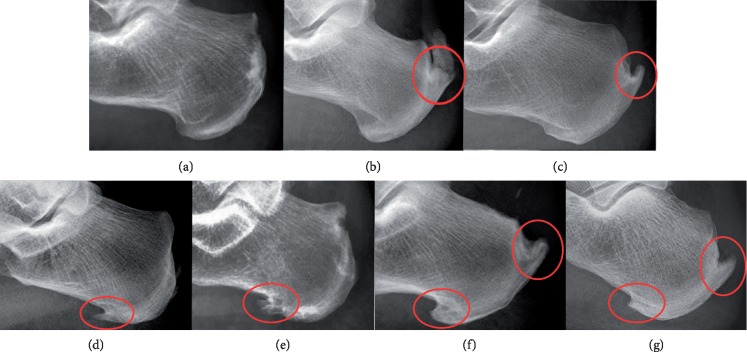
Examples of X-ray obtained in the study. (a) no plantar calcaneal or posterior calcaneal spur; (b, c): posterior calcaneal spurs only; (d, e): plantar calcaneal spurs only; (f, g): spurs on bilateral sides of the calcaneus.
